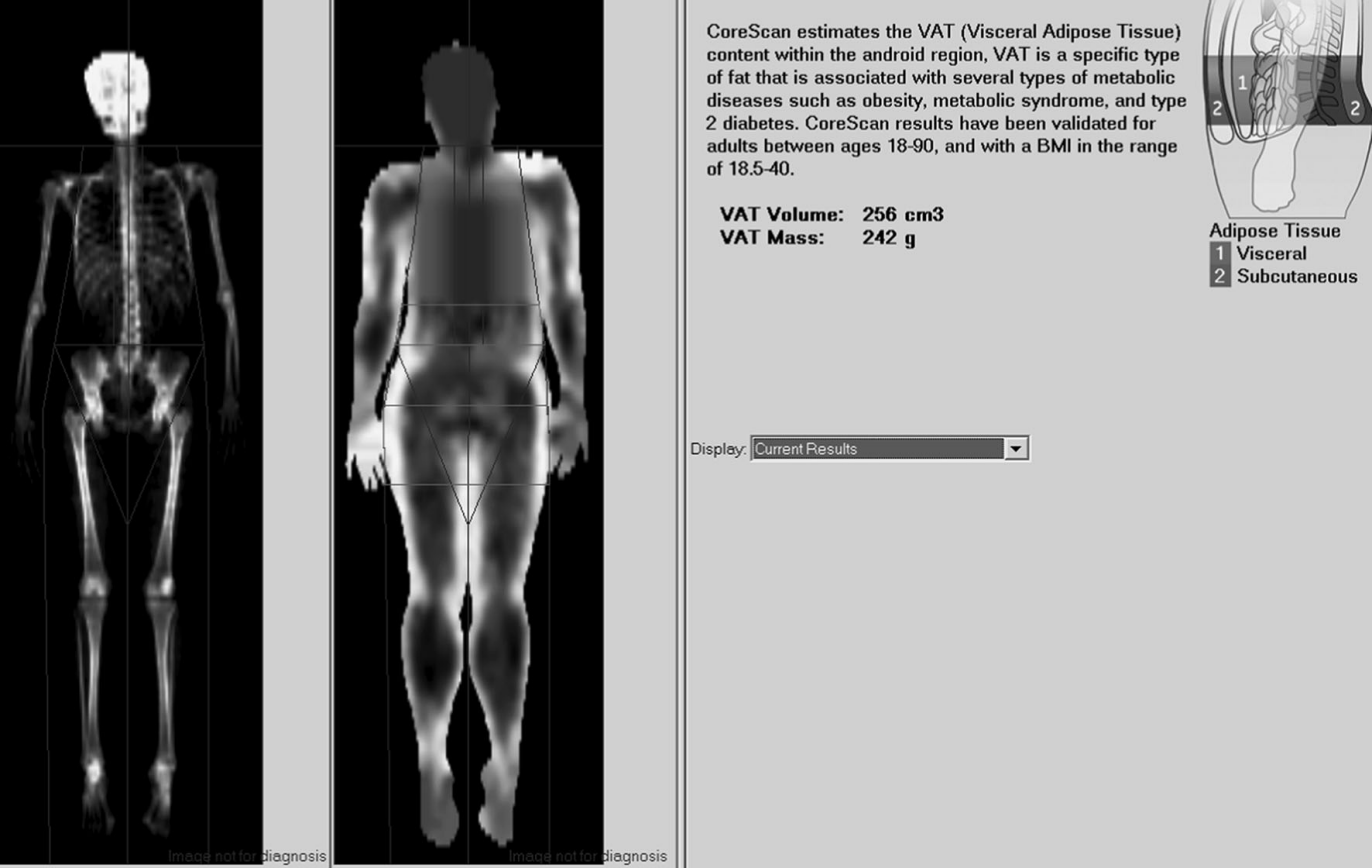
The assessment of visceral and SAT in the android region of interest by dual-energy X-ray absorptiometry. Total body scan of the study subject
